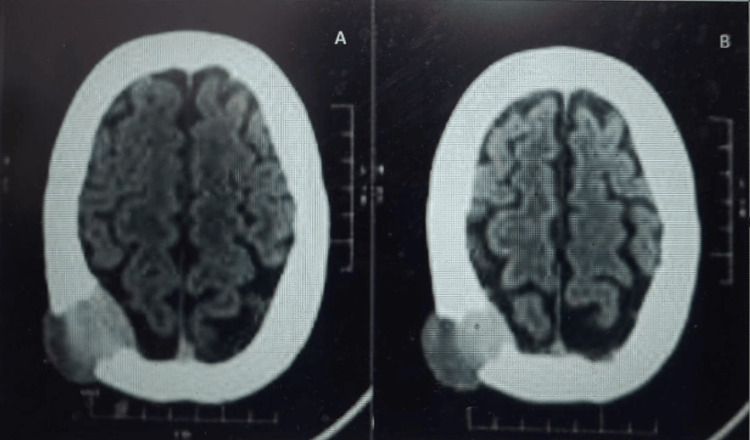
Computed tomography (CT) scan of the brain A, B: CT scan of the brain revealed a right parietal osseous lesion with calvarial destruction (which is located at approximately the 7 o’clock position in each image); the lesion was consistent with hematogenous metastasis from thyroid carcinoma.
